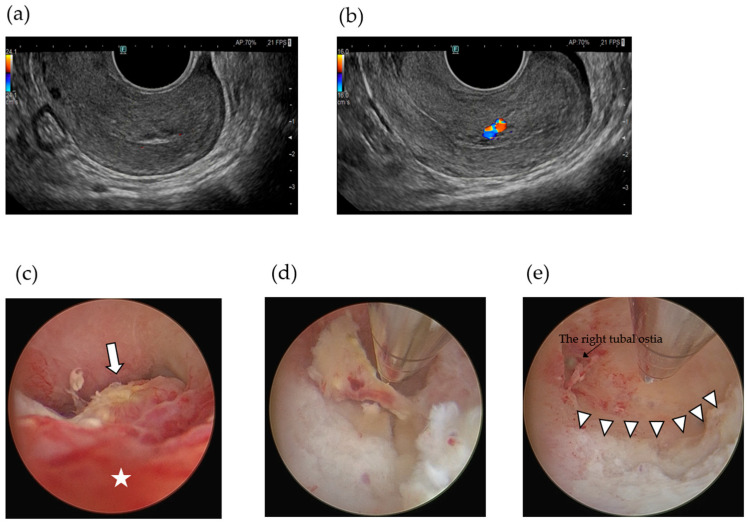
Transvaginal ultrasonography showing a hypovascular mass in the endometrium after uterine artery embolization (a). On day 17 after UAE, partial revascularization of the retained products of conception (RPOC) was observed on Doppler ultrasound (b). Hysteroscopic view showing two distinct areas of RPOC: a hyperemic, reddish lesion indicating revascularization (white star), and a whitish, shrunken area representing devascularized tissue (arrow) (c). Complete resection of the RPOC was achieved using a hysteroscopic morcellation system (triangles) (d,e).
